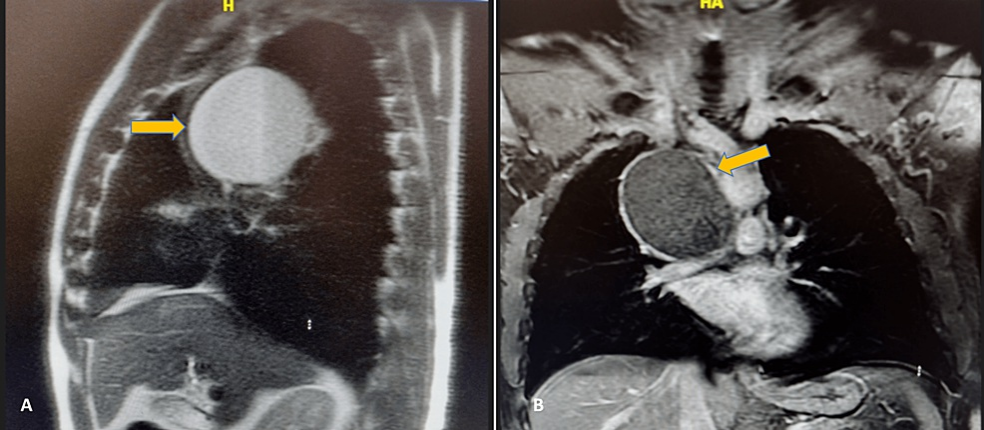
(A) Sagittal view of MRI of the chest. (B) Coronal view of MRI of the chest
